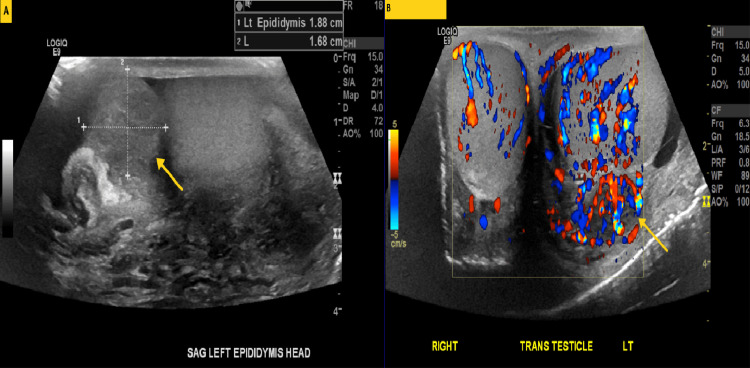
Representative grayscale (A) and color doppler (B) sonographic images through the left epididymis/testicle demonstrate enlarged and hypervascular left testicle and epididymis suggestive of epididymo-orchitis.
